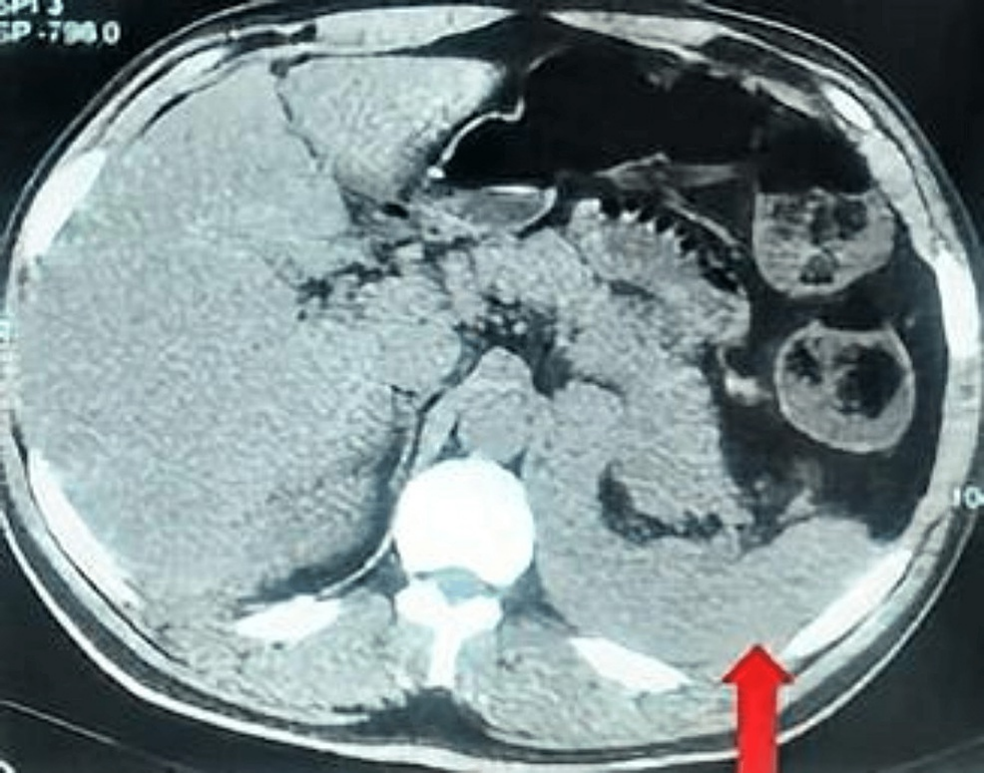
A contrast CT abdomen transverse view showing a recurrence of the tumour in the left adrenal bed (arrow).
